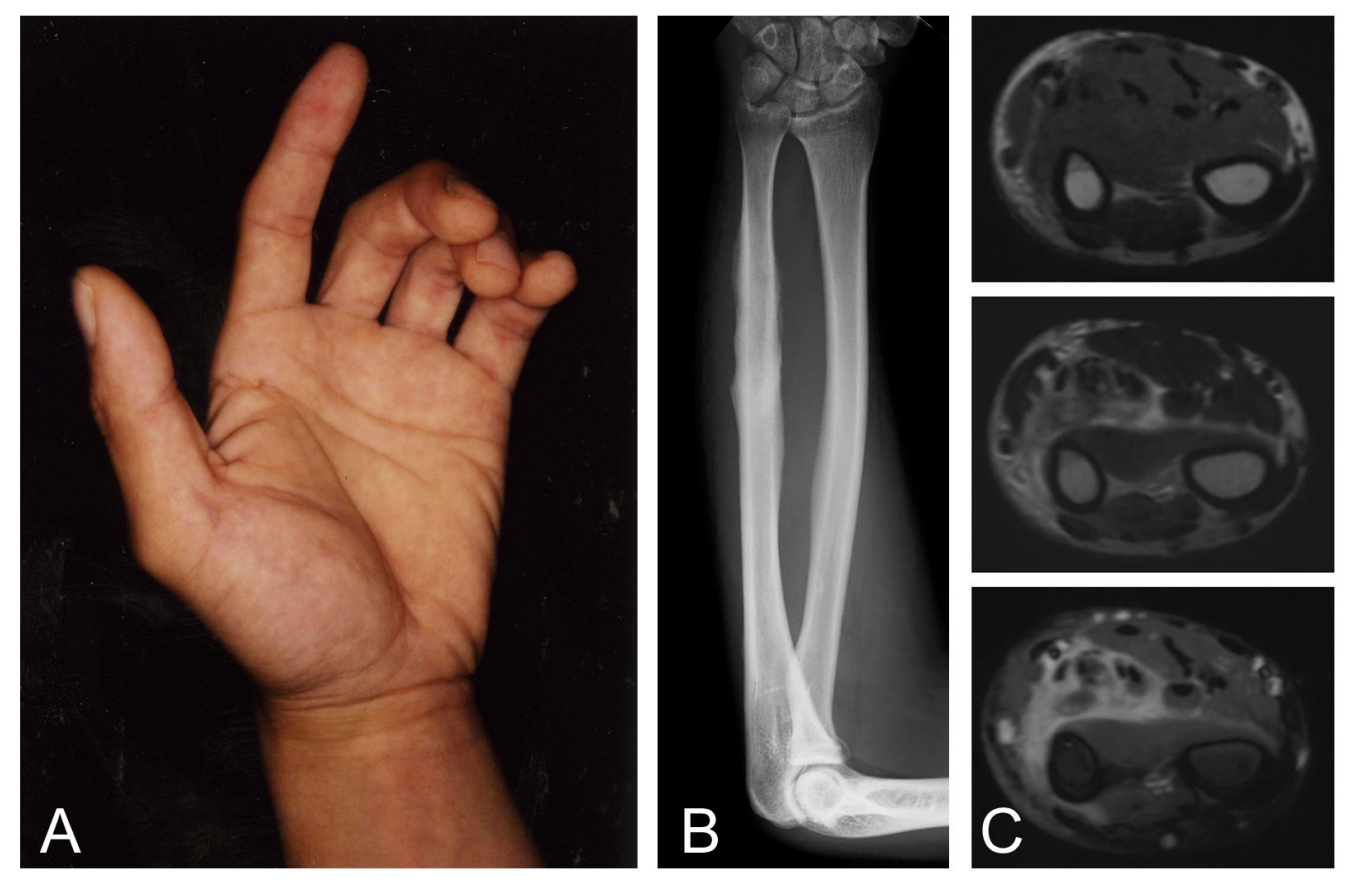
Epithlioid sarcoma in the forearm. Flexion contracture of the fingers can be seen (A). Plain radiograph shows irregular surface of the ulna (B). MRI of the forearm shows an abnormal lesion with iso-signal intensity on T1-weighted image (top) and high-signal intensity on T2-weighted image (middle) (C). Enhancement with gadolinium can be seen on T1-weighted fat-suppression image (bottom) (C).
